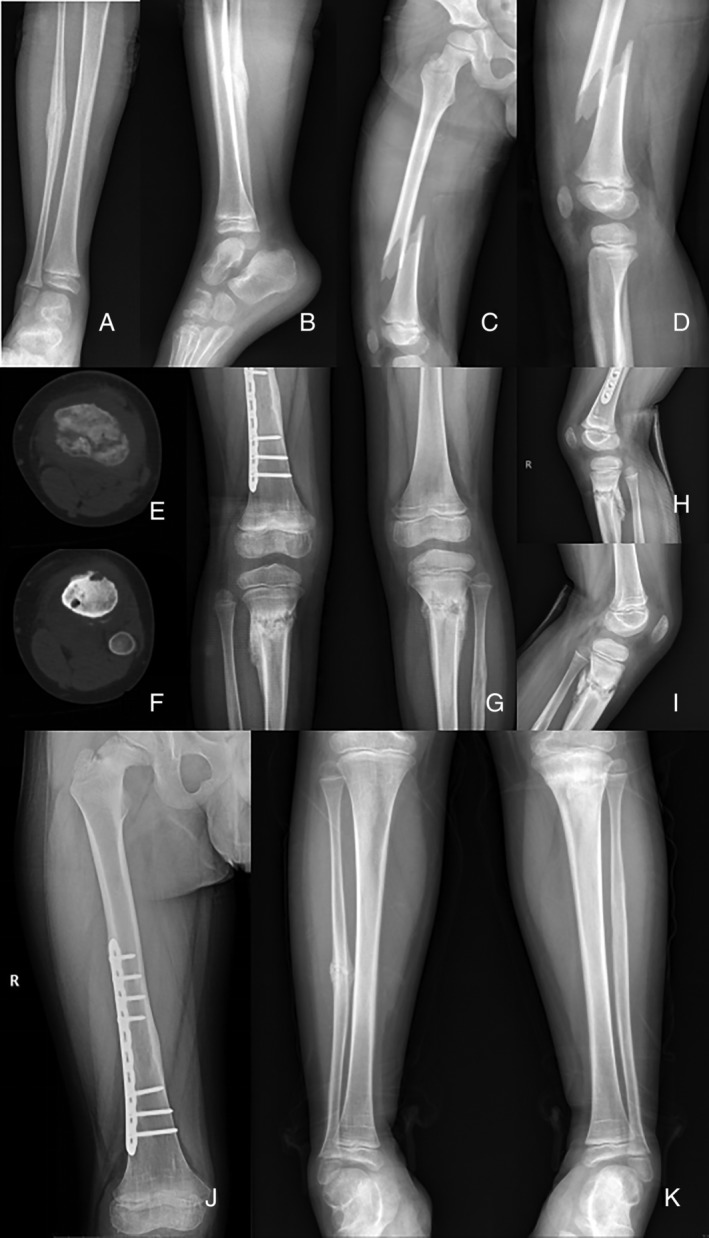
(A,B) Old fracture of the middle part of the right fibula, eschar formation (X‐ray positive and lateral position). (C,D) Right middle and lower thigh fracture, spiral fracture line (X‐ray positive and lateral position). (E,F) Bilateral proximal tibia fractures, CT showed cavity formation in the bone marrow cavity. (G,H,I) Bilateral proximal tibia fractures, the fracture site showed a large number of eschar formation (X‐ray positive and lateral position). (J) The old fracture of the right thigh neck, the shortening of the neck of the thigh. (K) Old fracture of the middle part of the fibula on the right, scab formation.
